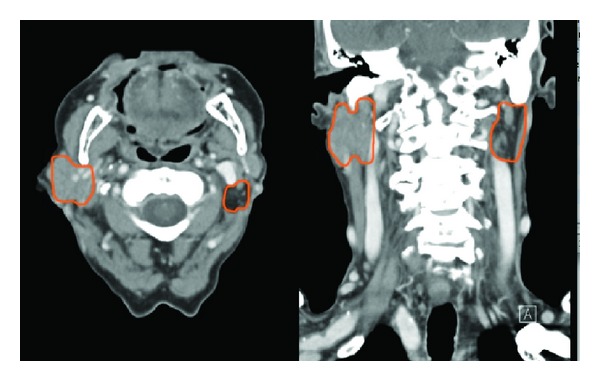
Head and neck CT scan (axial and coronal views) made 4 years after the left parotidectomy, showing a moderate partial atrophy of the autologous fat graft.
